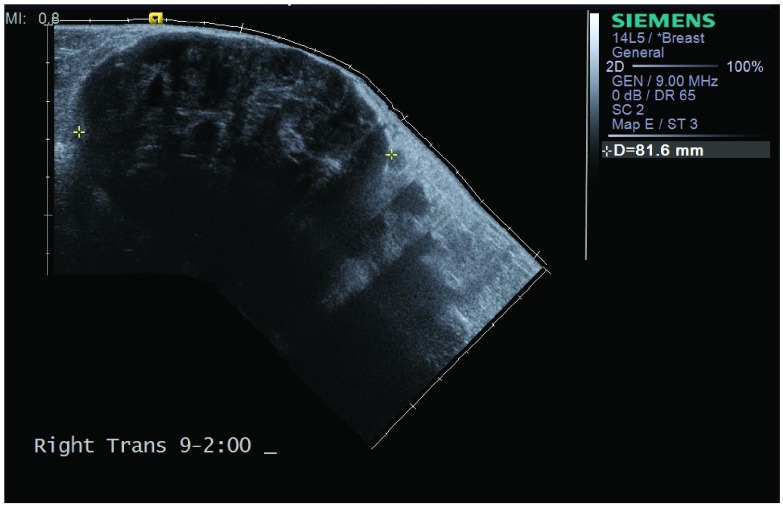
Right breast ultrasound demonstrates a large heterogeneous primarily hypoechoic lobulated mass encompassing most of the right breast.
